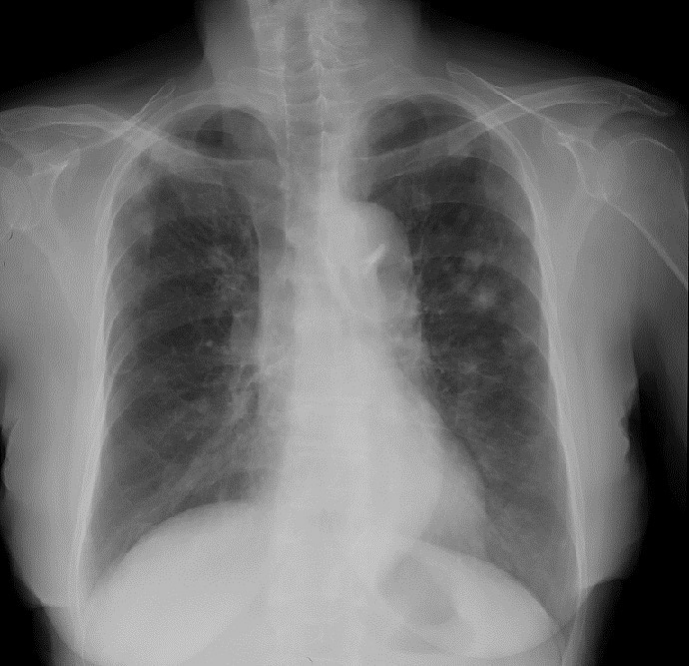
A Chest radiograph performed at the time of presentation showing multiple nodules bilaterally that are predominantly in the upper lung fields.
